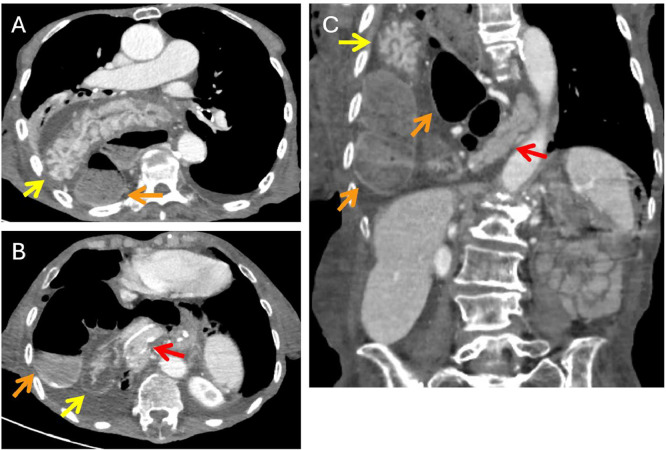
Axial (A, B) and coronal reconstruction (C) of thoraco-abdominal CT scan show herniated abdominal content including stomach (yellow arrows), colonic loops (orange arrows) and body and tail of the pancreas (red arrows) along with mesenteric fat and vessels consistent with diaphragmatic hernia. Note the dilated colonic bowel loops and the presence of free fluid related to chronic distal large bowel obstruction.
